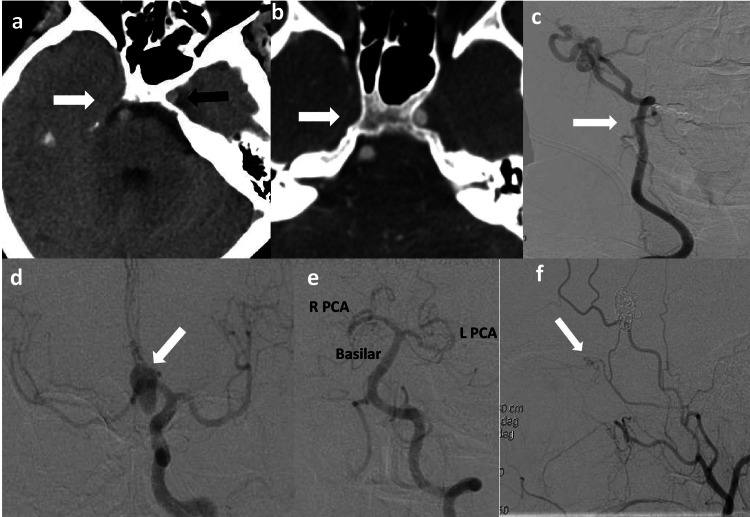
(a) CT head shows the absence of the right carotid canal (white arrow) and the well-formed left carotid canal (black arrow). (b) CT angiogram head shows the absence of right ICA (white arrow). (c) Right common carotid injection (AP view) reveals the absence of right ICA and opacification of ECA (white arrow). (d) Left common carotid injection (AP view) shows the large ACOM aneurysm (white arrow) with bilateral MCA’s and ACA’s being supplied by Left ICA. (e) Left vertebral artery injection (AP view) shows basilar and bilateral posterior cerebral arteries (PCA), but did not reveal any collaterals from posterior to anterior circulation. (f) Left common carotid injection (Lateral view) shows an ophthalmic artery (white arrow) coming off the MMA. CT: computed tomography; ICA: internal carotid artery; AP: anterior-posterior; ECA: external carotid artery; ACOM: anterior communicating artery; MCA: middle cerebral artery; ACA: anterior cerebral artery; PCA: posterior cerebral artery; MMA: middle meningeal artery
